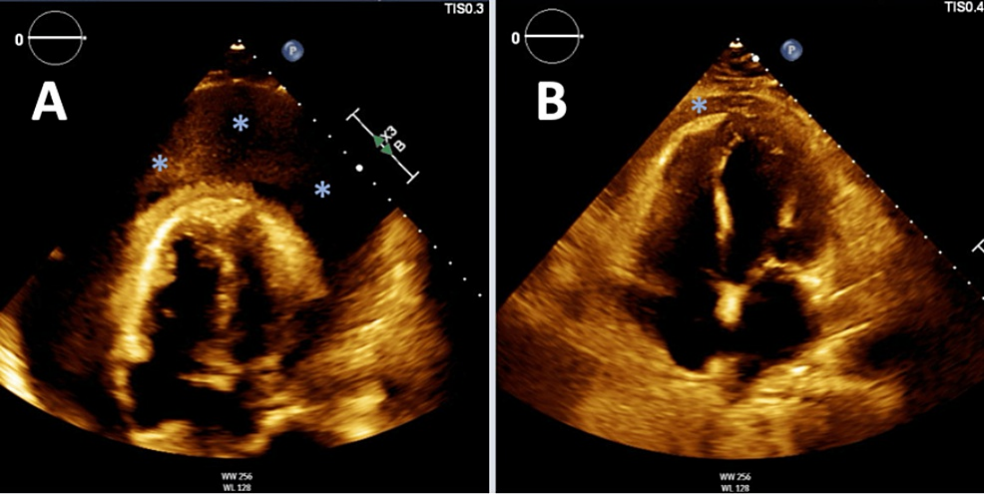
Transthoracic echocardiogram apical four-chamber view (A) TTE on presentation showing large pericardial effusion with impending tamponade (asterixis). (B) TTE following pericardiocentesis showing trace pericardial effusion (asterixis).
